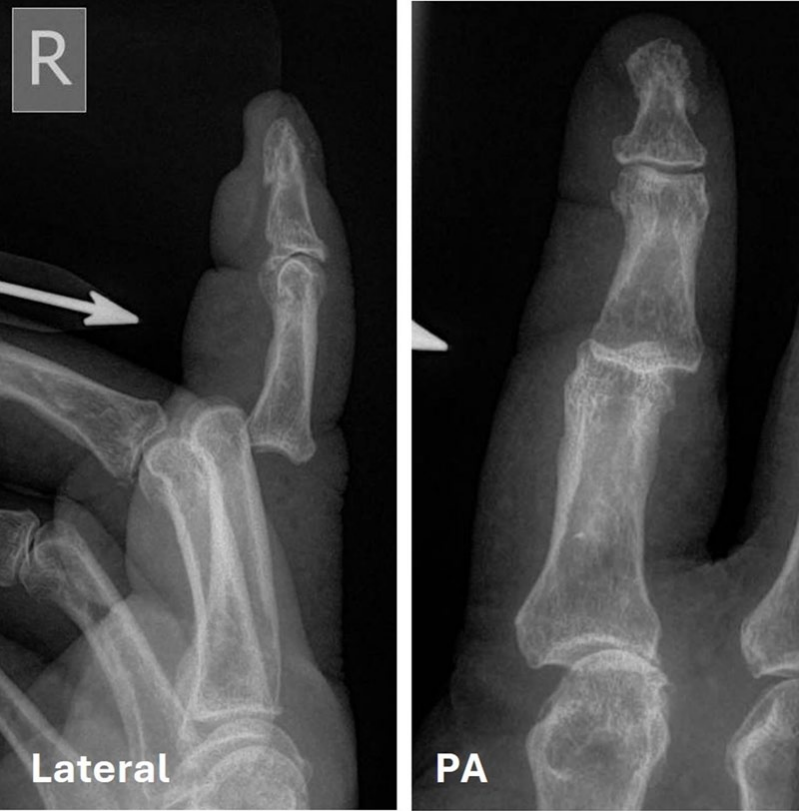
Radiograph showing an open dorsal dislocation of the index finger. Half (3/6) of all index finger dislocations were open.
